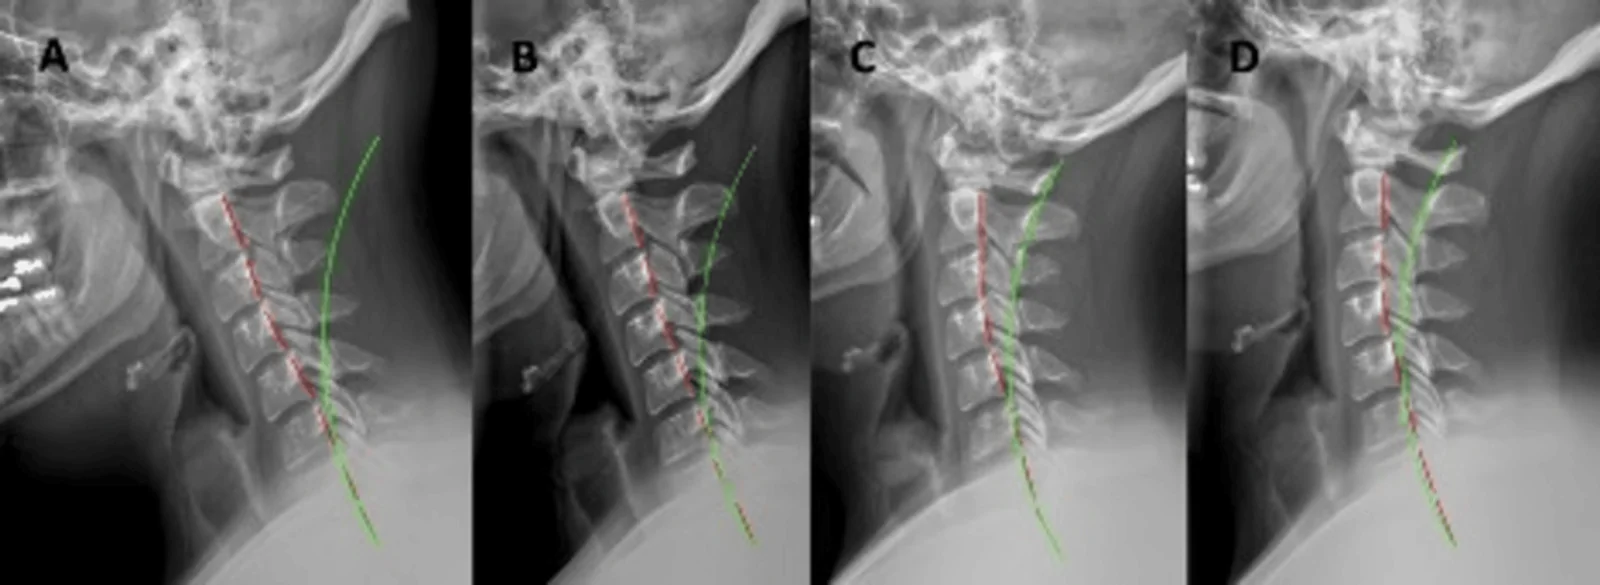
Lateral cervical radiographs: (A) pre-treatment, (B) first post-treatment, (C) second post-treatment, and (D) 17-month follow-up.
